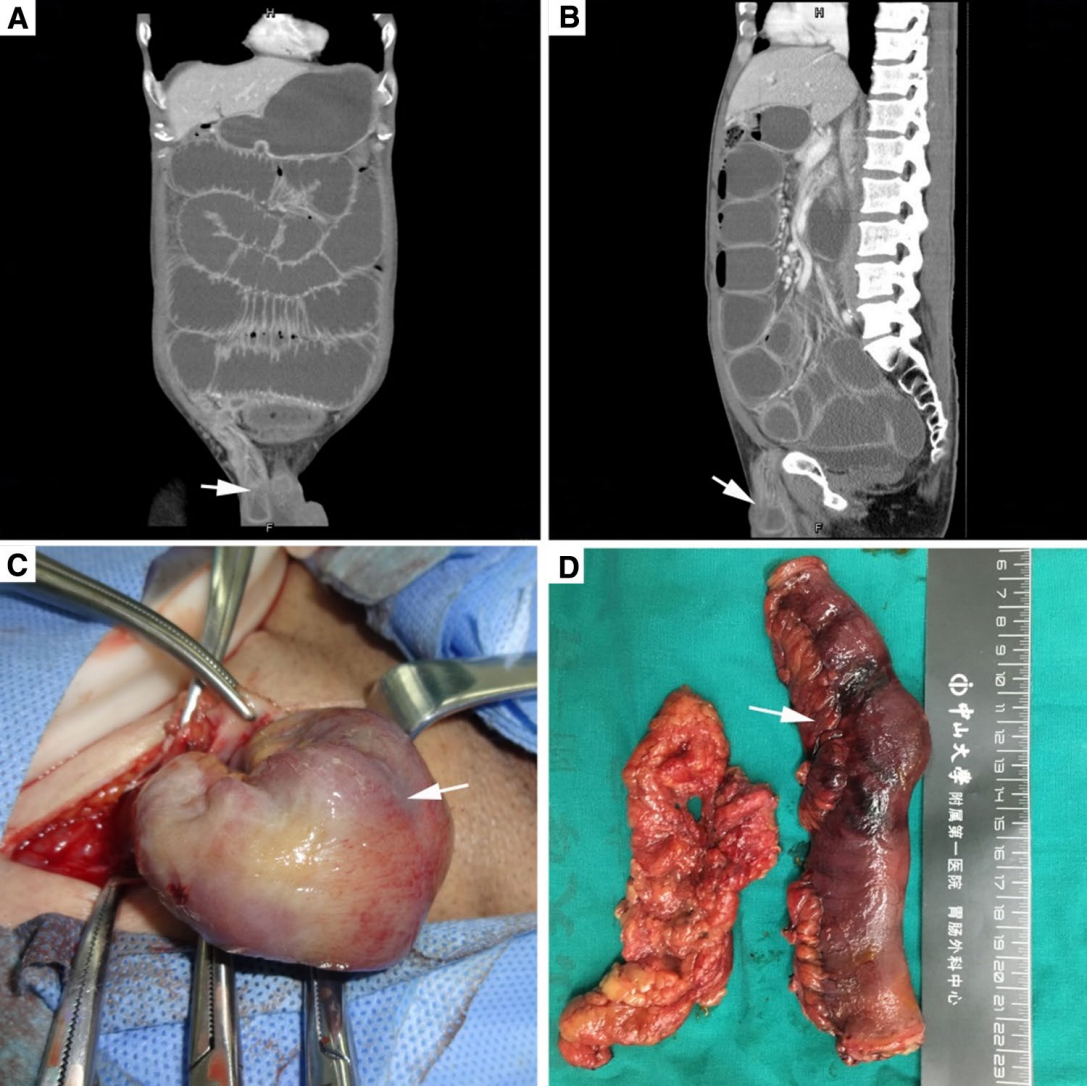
Demonstration of surgical management of incarcerated groin hernia. This was a 46-year-old male patient who presented with severe groin pain and abdominal distension for 10 h. a Coronal view of abdominal CT scan; b sagittal view of abdominal ct scan; c surgical exploration from the groin incision; d resected bowel and greater omentum strangulated in the right groin area. The white arrows in both a and b show the strangulated inguinal hernia on the right-sided groin. In addition, the patient has intestinal obstruction. White arrow heads (c and d) refer to intestinal necrosis
